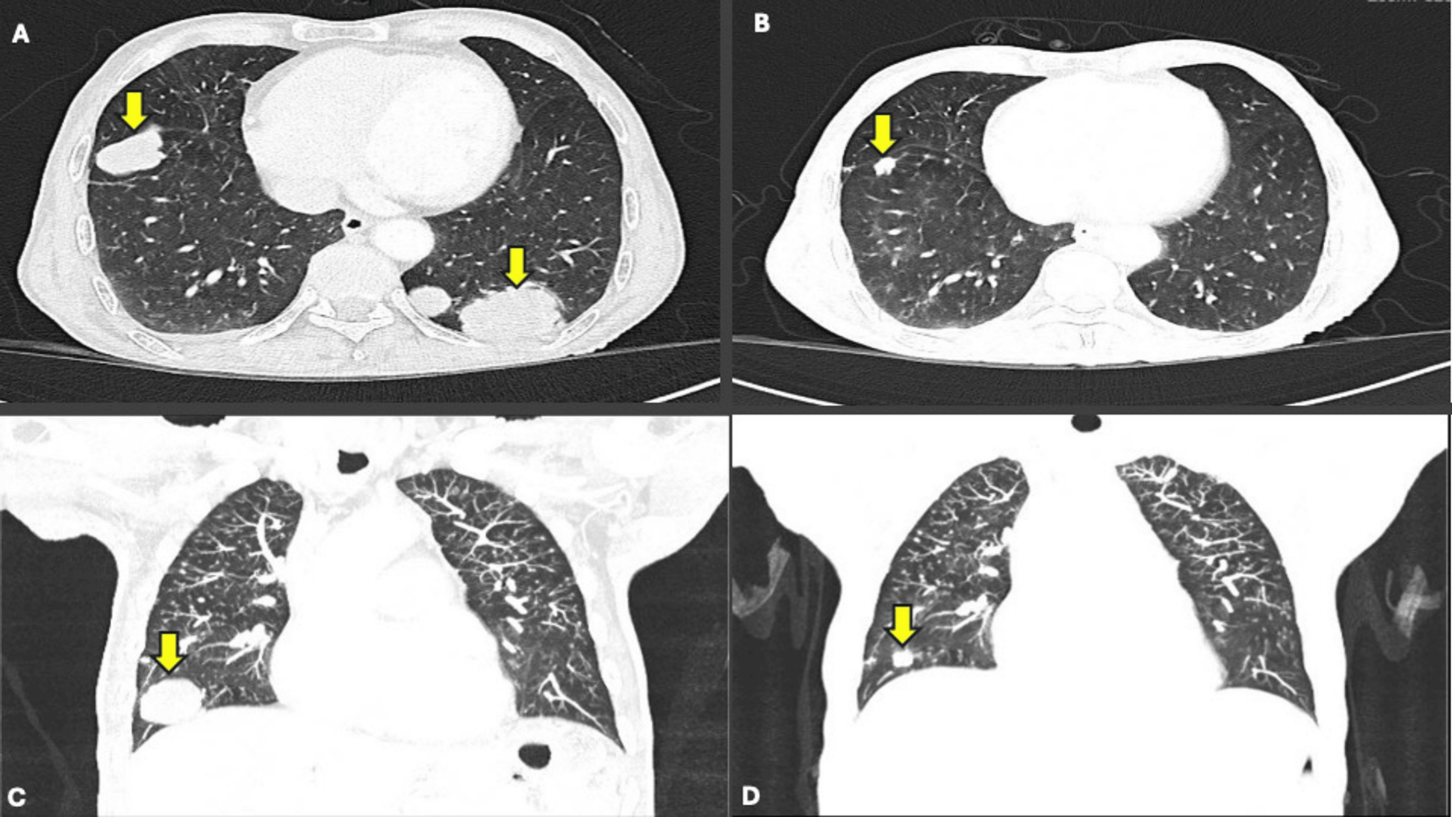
Thoracic CT scans before and after four cycles of chemoimmunotherapy (A) Baseline axial CT scan from January 2024 showing the two largest pulmonary metastases (yellow arrows) in the posterior segment of the right lower lobe and the posterior basal segment of the left lower lobe. (B) Follow-up axial CT scan from July 2024 demonstrating a marked reduction in the size of both metastases (yellow arrows), indicating a partial radiologic response. No new metastases or infiltrative changes are observed. (C) Baseline coronal CT scan from January 2024 confirming the two largest pulmonary metastases (yellow arrows) in the right and left lower lobes. (D) Follow-up coronal CT scan from July 2024 showing a significant reduction in the size of both metastases (yellow arrows), with no new metastases or infiltrative changes.
